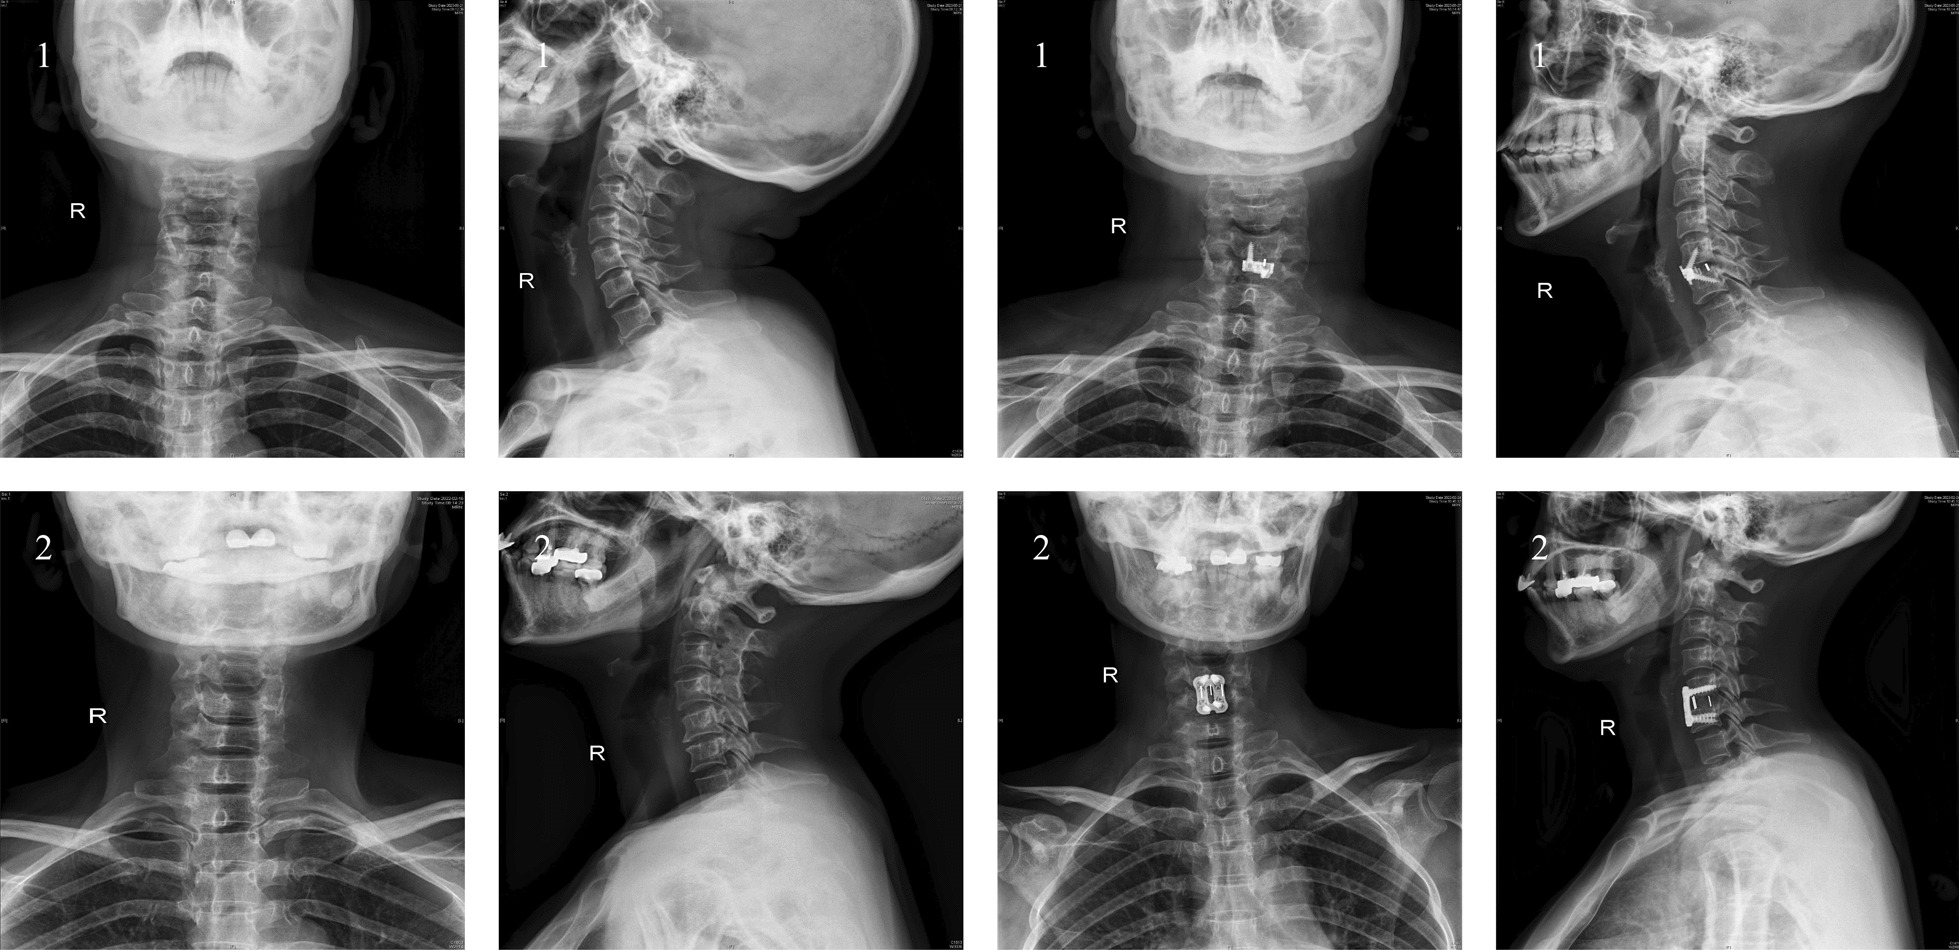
Postoperativel coronal and sagittal cervical X-ray with Zero-P or Cage-plate surgery. 1A, 1B Postoperative cervical X-ray with Zero-p surgery. 1C, 1D X-ray at postoperative 1 month with Zero-p surgery. 2A, 2B Postoperative cervical X-ray with Cage-plate surgery. 2C, 2D X-ray at postoperative 1 month with Cage-plate surgery
